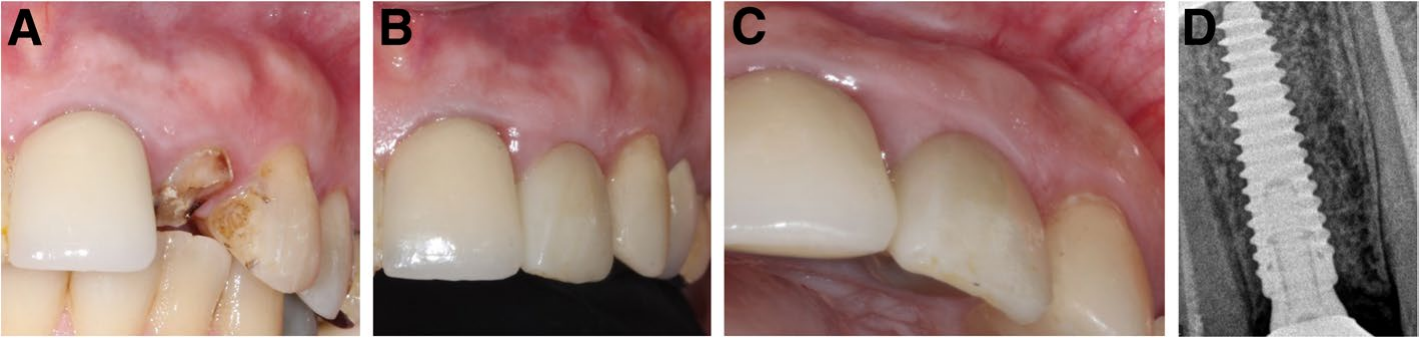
A-D: Photos and a radiograph of case #2 replacing the maxillary left lateral incisor. a Preoperative frontal view; b frontal view of the definitive crown at 12 months postloading; c incisal view of the definitive crown at 12 months postloading; and d periapical radiograph at 12 months postloading
